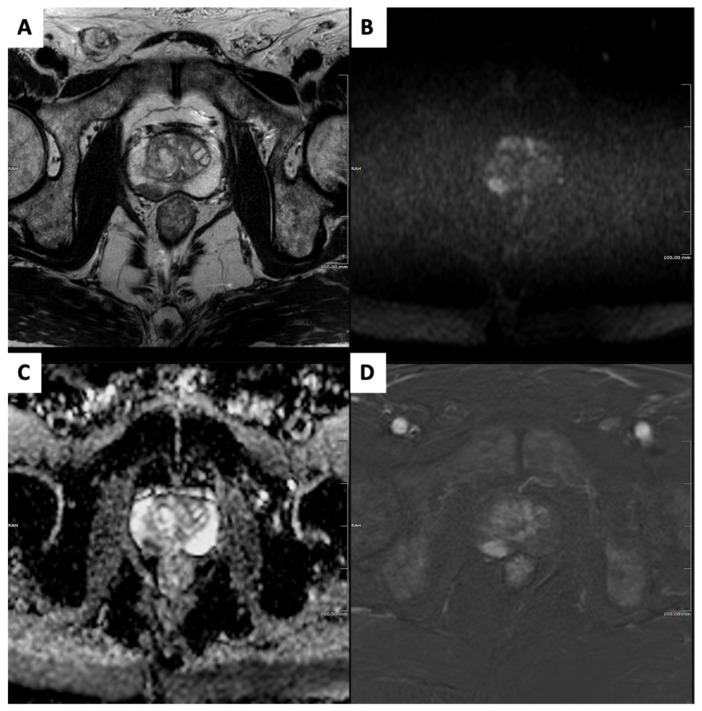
Clinically significant prostate cancer in a 75-year-old biopsy-naïve subject who underwent magnetic resonance imaging (MRI) for raised prostatic-specific antigen level (7 ng/mL) and suspicious digital rectal examination. Prostate MRI showed a 13 mm PI-RADSv2.1 category 4 focus in the right mid-gland peripheral zone, demonstrating homogeneous moderate hypointensity on axial T2-weighted image (A); focal-restricted diffusion with marked hyperintensity on the high b-value image (B) and marked hypointensity on the apparent diffusion coefficient map (C); and focal, early enhancement on a digitally subtracted, fat-saturated T1-weighted image from the dynamic contrast-enhanced sequence (D). Targeted cores from transperineal biopsy showed a ISUP grade 2 prostate cancer.
